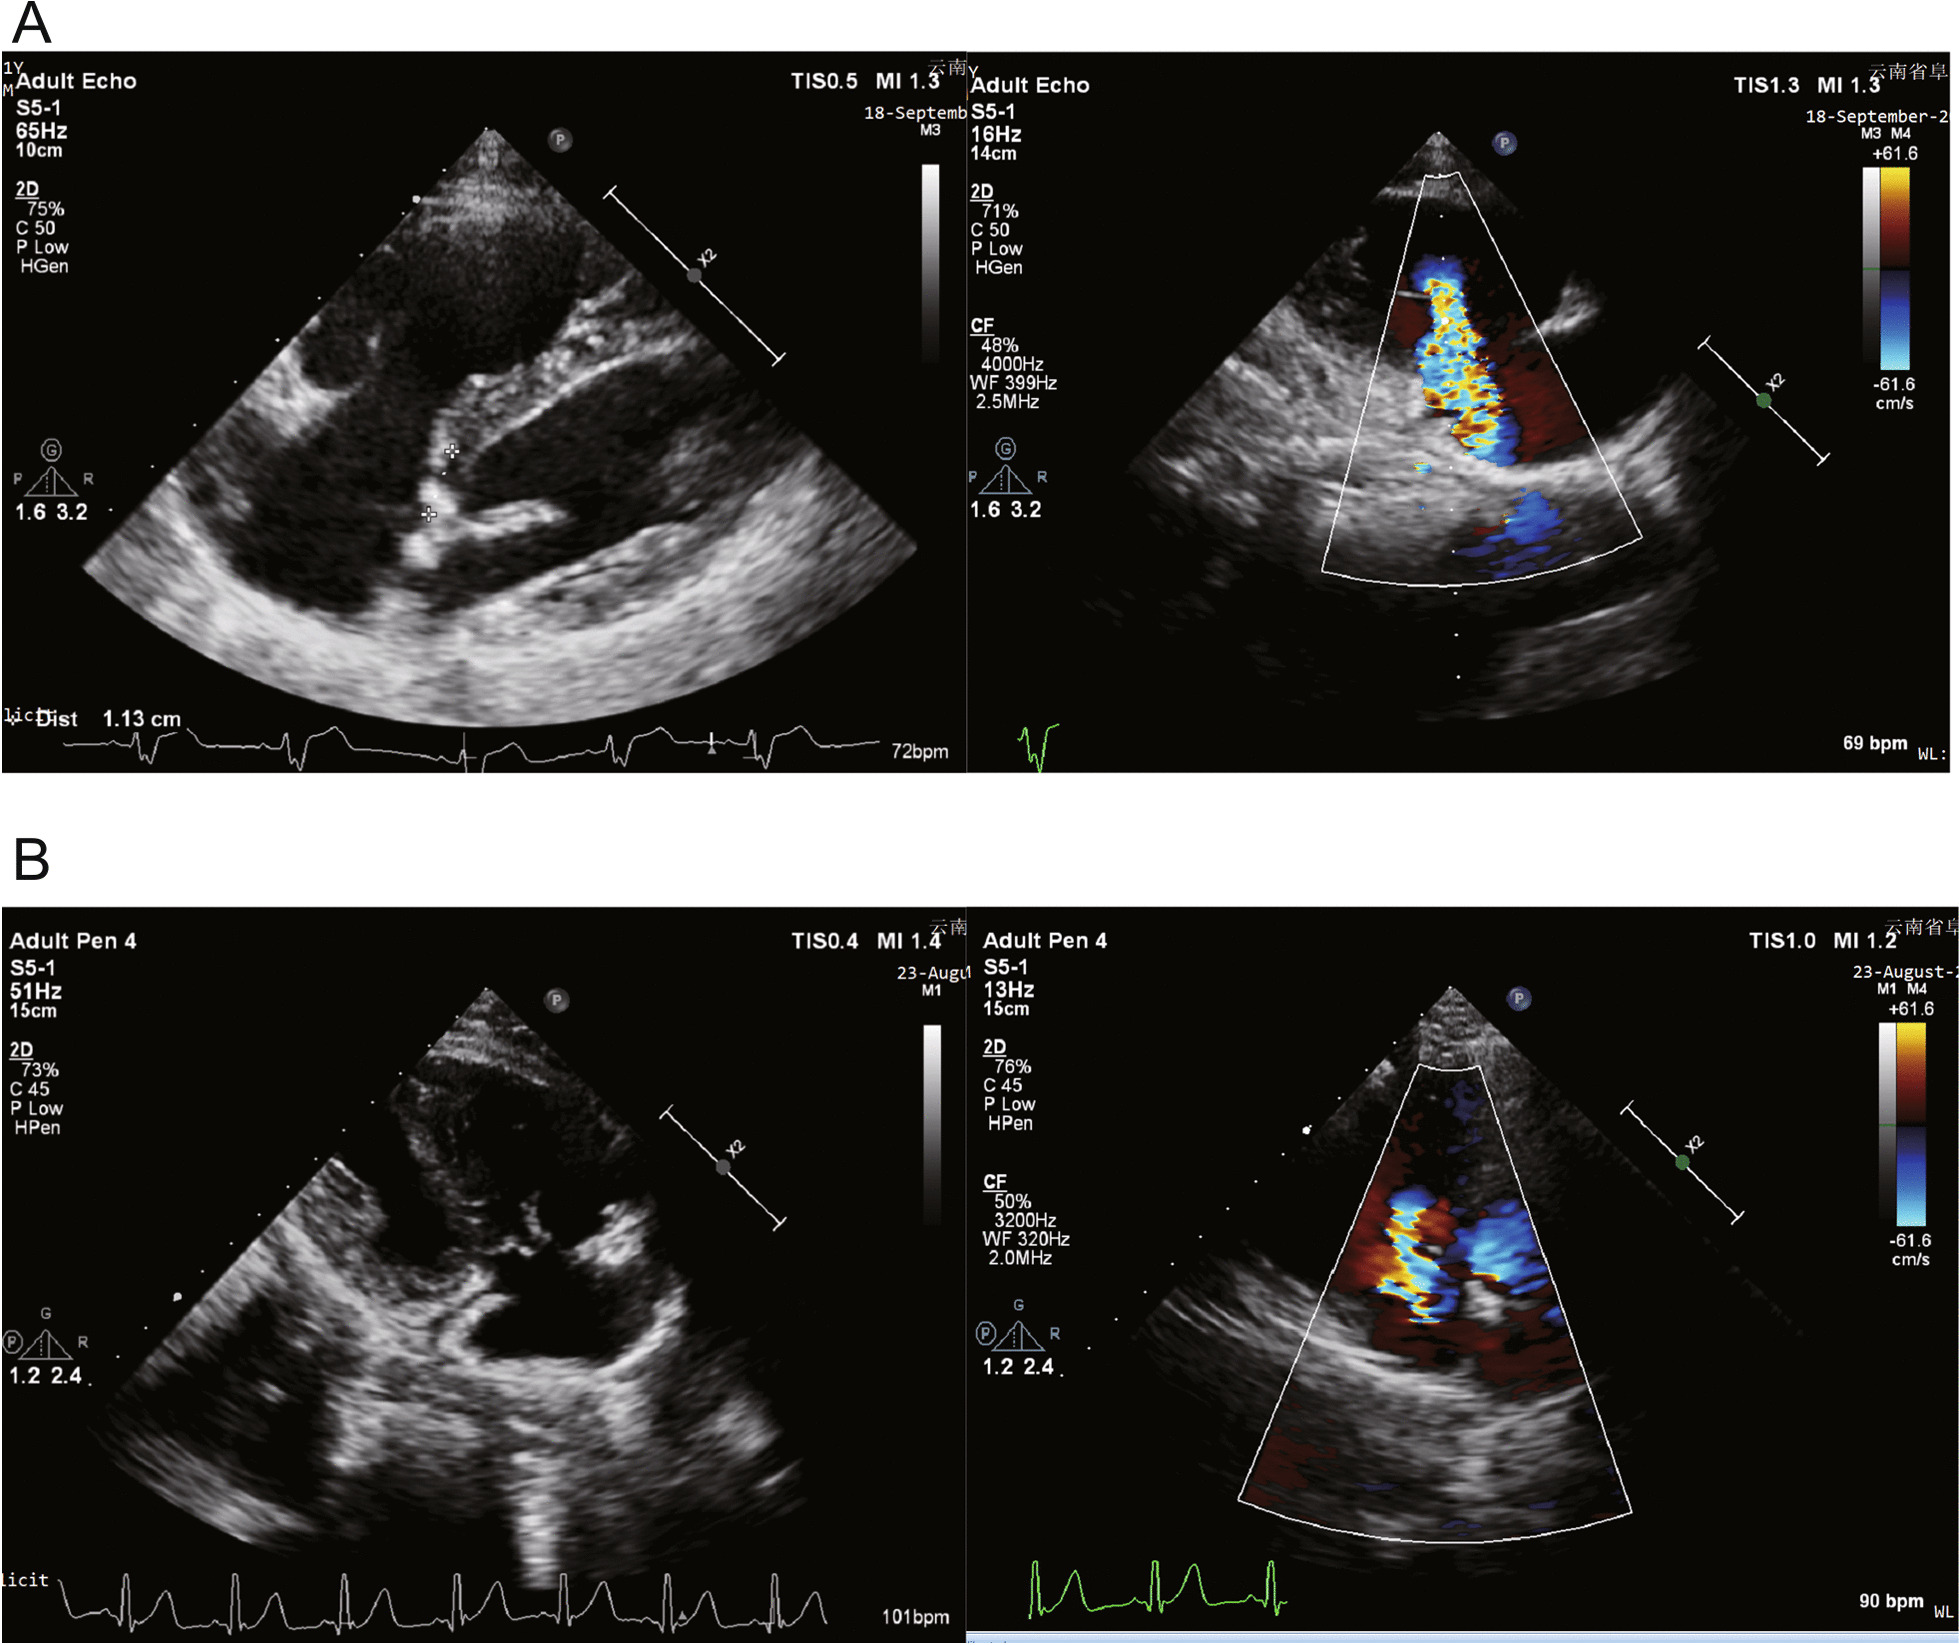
a The oblique four-chamber section of transthoracic echocardiography of Twin 1 shows that the septal lobe of the tricuspid valve moves downward to the apical part of the heart by transthoracic echocardiography, and the right ventricle inflow tract section by transthoracic echocardiography shows a large volume of tricuspid regurgitation by colour Doppler. b The oblique four-chamber section by transthoracic echocardiography of Twin 2 shows that the septal lobe of the tricuspid valve protrudes slightly into the right atrium during systole; the colour Doppler shows tricuspid regurgitation in the inflow section of the right ventricle by transthoracic echocardiography
